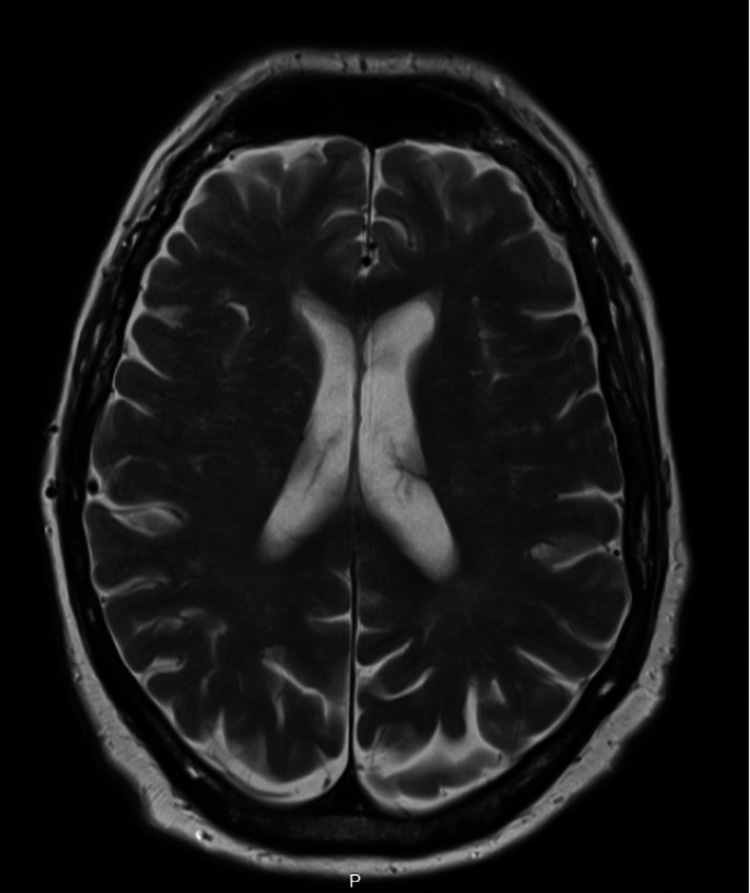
T2-weighted axial MRI of the brain image The MRI of the brain image showed no space-occupying lesion or brain metastasis as possible causes for the patient's symptoms. MRI: magnetic resonance imaging
